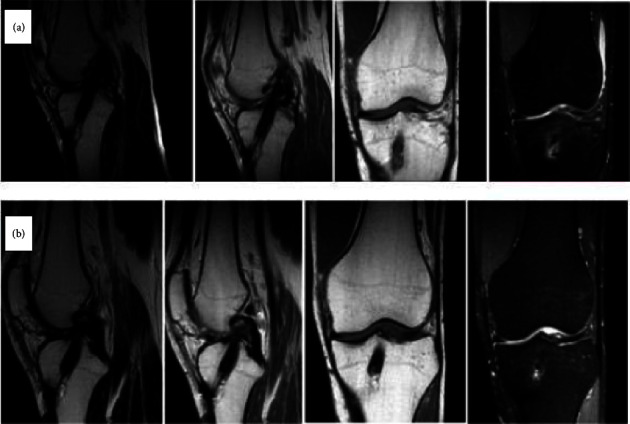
MRI graft images of a case during the 4th (a) and 12th (b) month follow-up.
